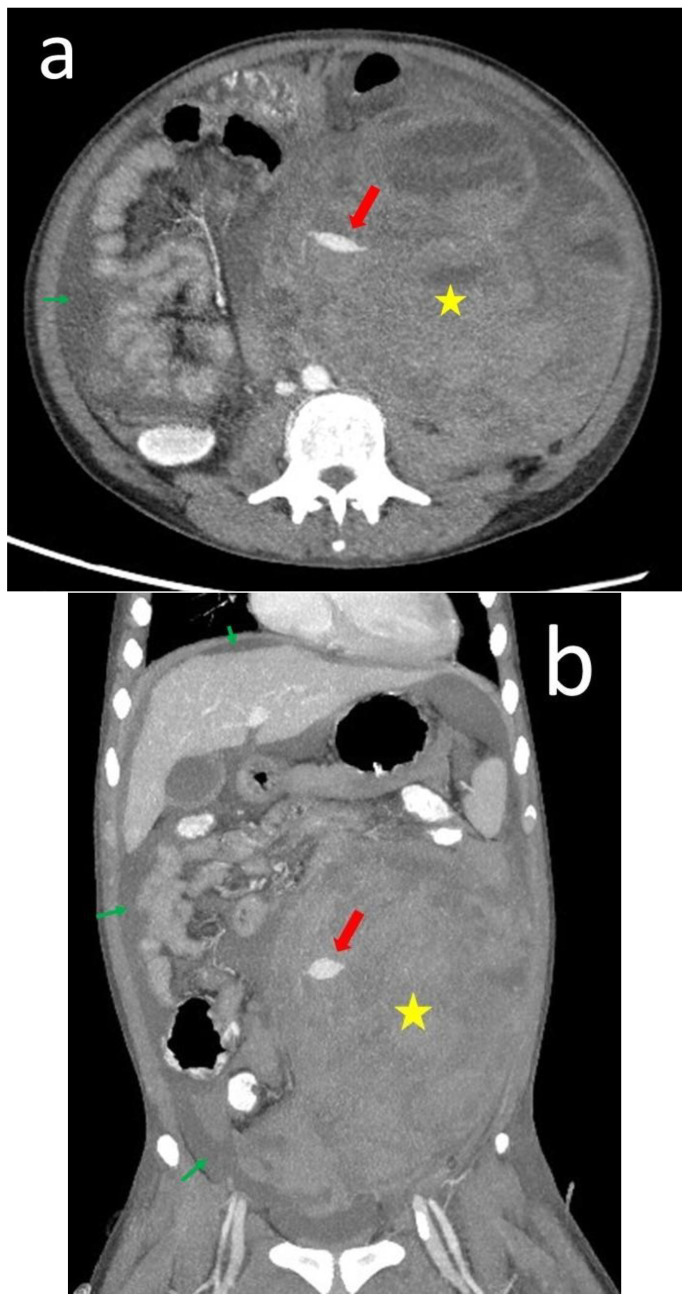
This is a figure showing the CT of the abdomen on day 5 of admission. Axial view (a) and coronal view (b). There was an active hemorrhage in the left retroperitoneal space hematoma (yellow asterisk) with high-density contrast retention (red arrow). The ascites increased compared with the day of admission (green thin arrow).
